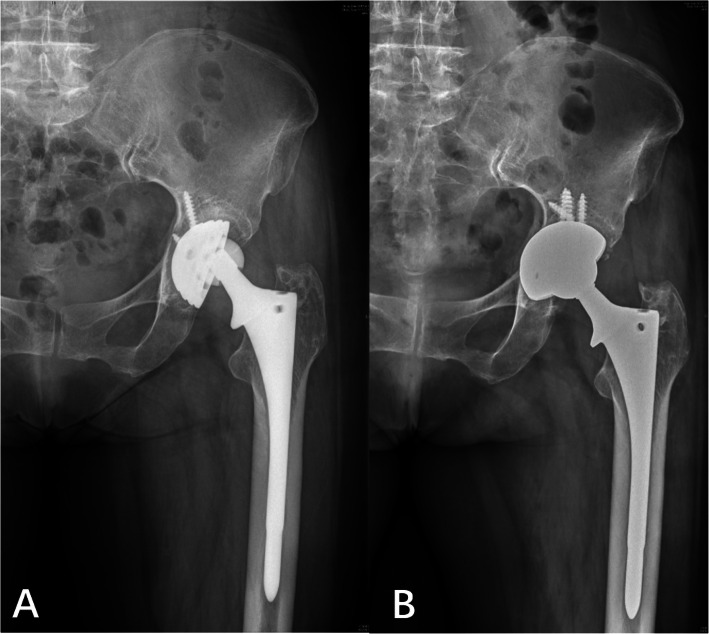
Postoperative radiographs of cylindrical stem with osteolysis around cup and re-revision with cup exchange
